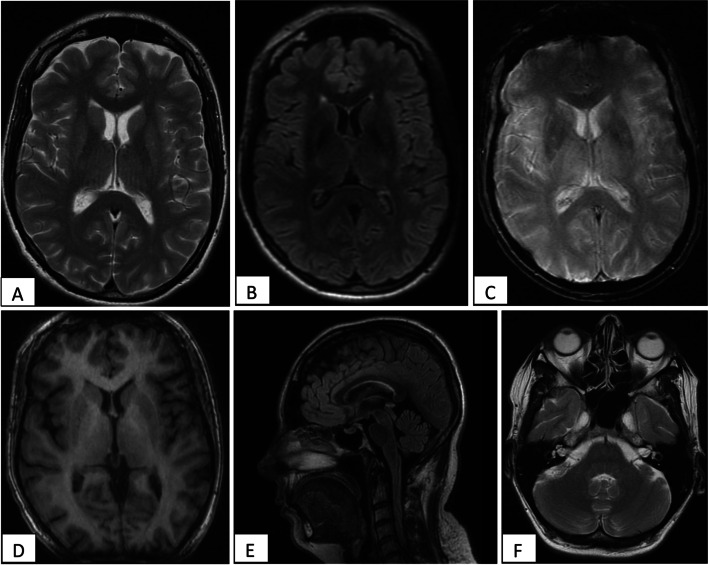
Brain Magnetic Resonance Imaging of the patient, revealing absence of abnormalities especially within basal ganglia. A Axial T2 sequencing centered on basal ganglia; B Axial FLAIR sequencing centered on basal ganglia; C Axial T2* centered on basal ganglia; D Axial T1 sequencing centered on basal ganglia; E Sagittal FLAIR sequencing centered on midbrain; F Axial T2 sequencing centered on cerebellum)
